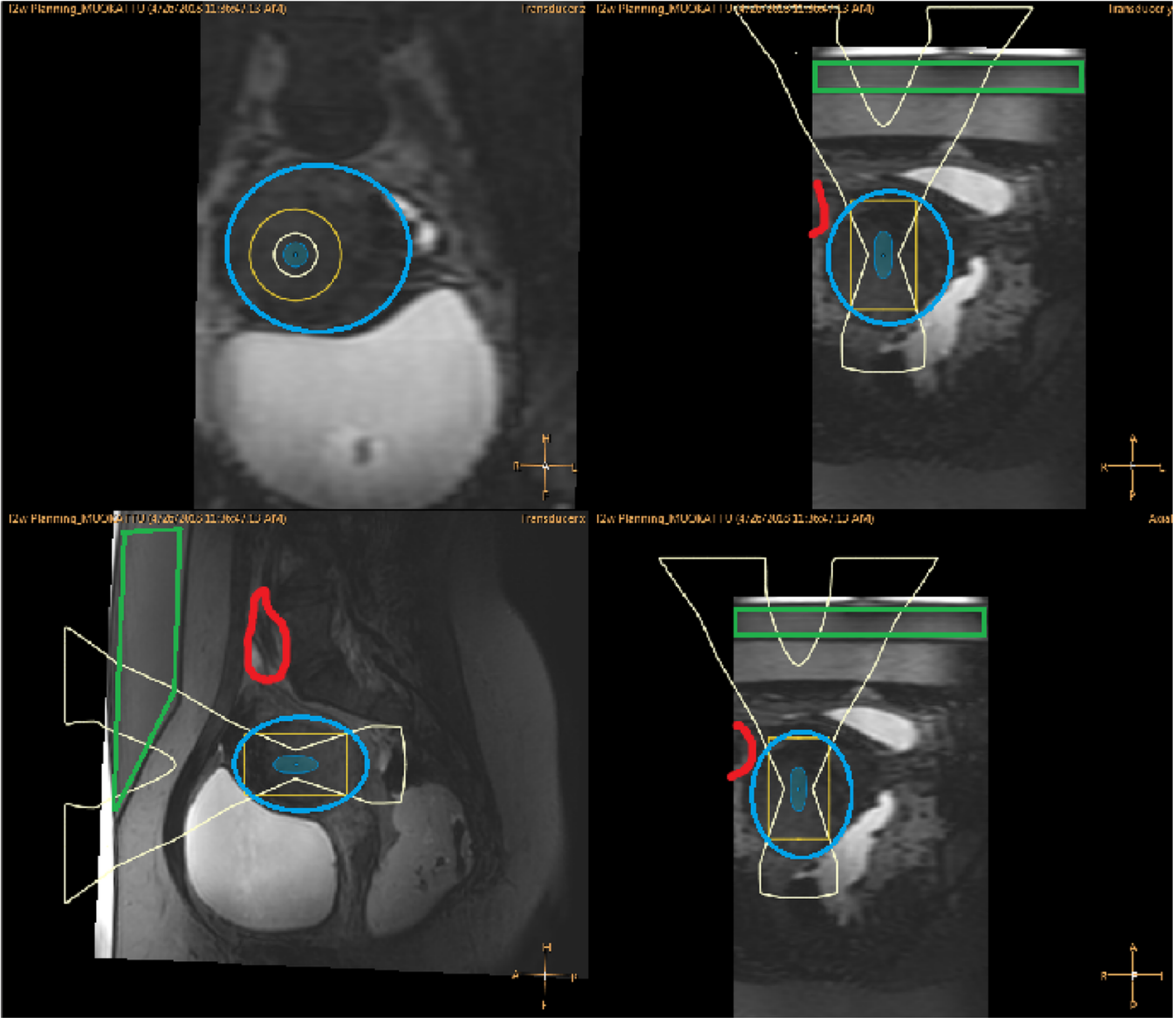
T2W planning images displayed on the therapy console with ultrasound beam view after application of wedged gel pad where red boundary is bowel, blue is uterine fibroid and green is the gel pad
